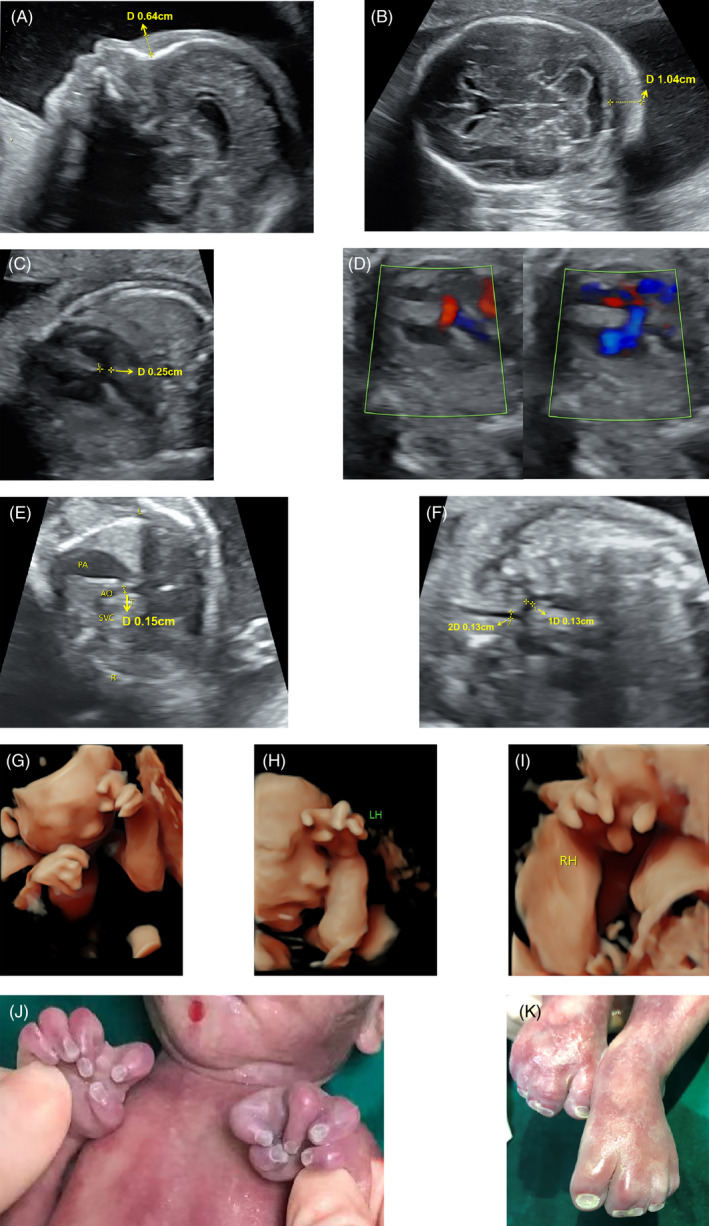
The ultrasound image of the fetus. (A) The anterior nasal skin is approximately 0.64 cm thick. (B) The nuchal fold is thickened to 1.04 cm. (C) The continuity of the ventricular septum is interrupted by approximately 0.25 cm. (D) Color Doppler flow imaging shows a bidirectional shunt on the ventricular level. (E) In the three‐vessel and trachea view, the ascending aorta is significantly narrower than the pulmonary artery. The transverse aortic arch is 0.15 cm wide. (F) The inner diameter of the aortic isthmus is 0.13 cm. (G–J) A wide gap between the thumb, index finger, and middle finger. Camptodactyly. Proximally placed fourth finger. (K) Syndactyly between second and third toes
